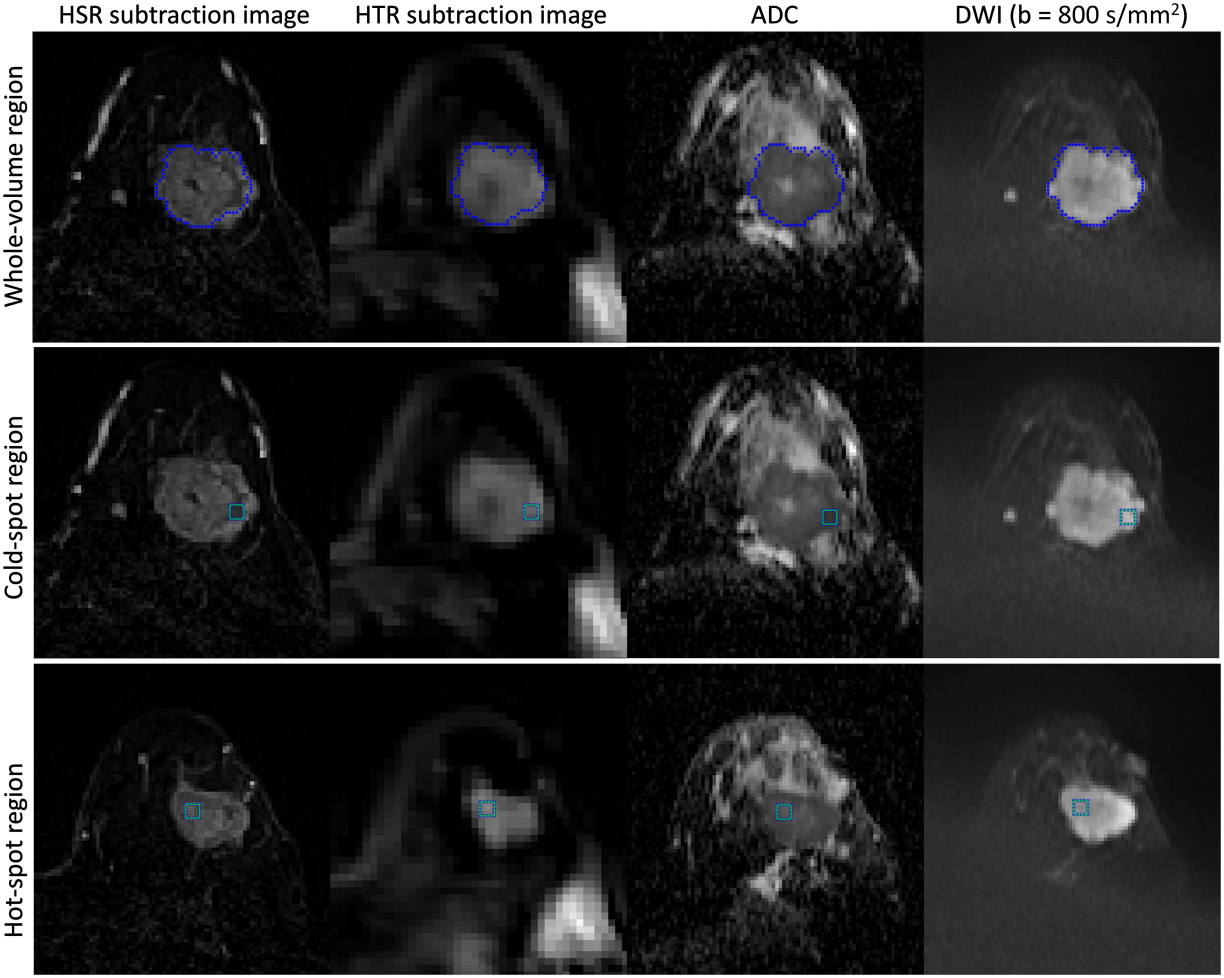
Example of seeding a tumor in a 45-year-old woman with invasive ductal carcinoma in the left breast and generating three regions of interest (whole-tumor, cold-spot, and hot-spot). First, the tumor was seeded on the HSR subtraction images, and the whole-tumor region of interest was generated (top row). Then, two smaller regions of interest (5×5 pixels) were generated within the whole-tumor region (cold-spot (middle row) and hot-spot regions (bottom row)). The whole-tumor region of interest encompasses all the slices in which the tumor appears, while the cold-spot and hot-spot regions originate in only a single slice (not necessarily the same slice). All three regions were propagated to the corresponding DWI, inversion recovery, and HTR images for further analysis.
